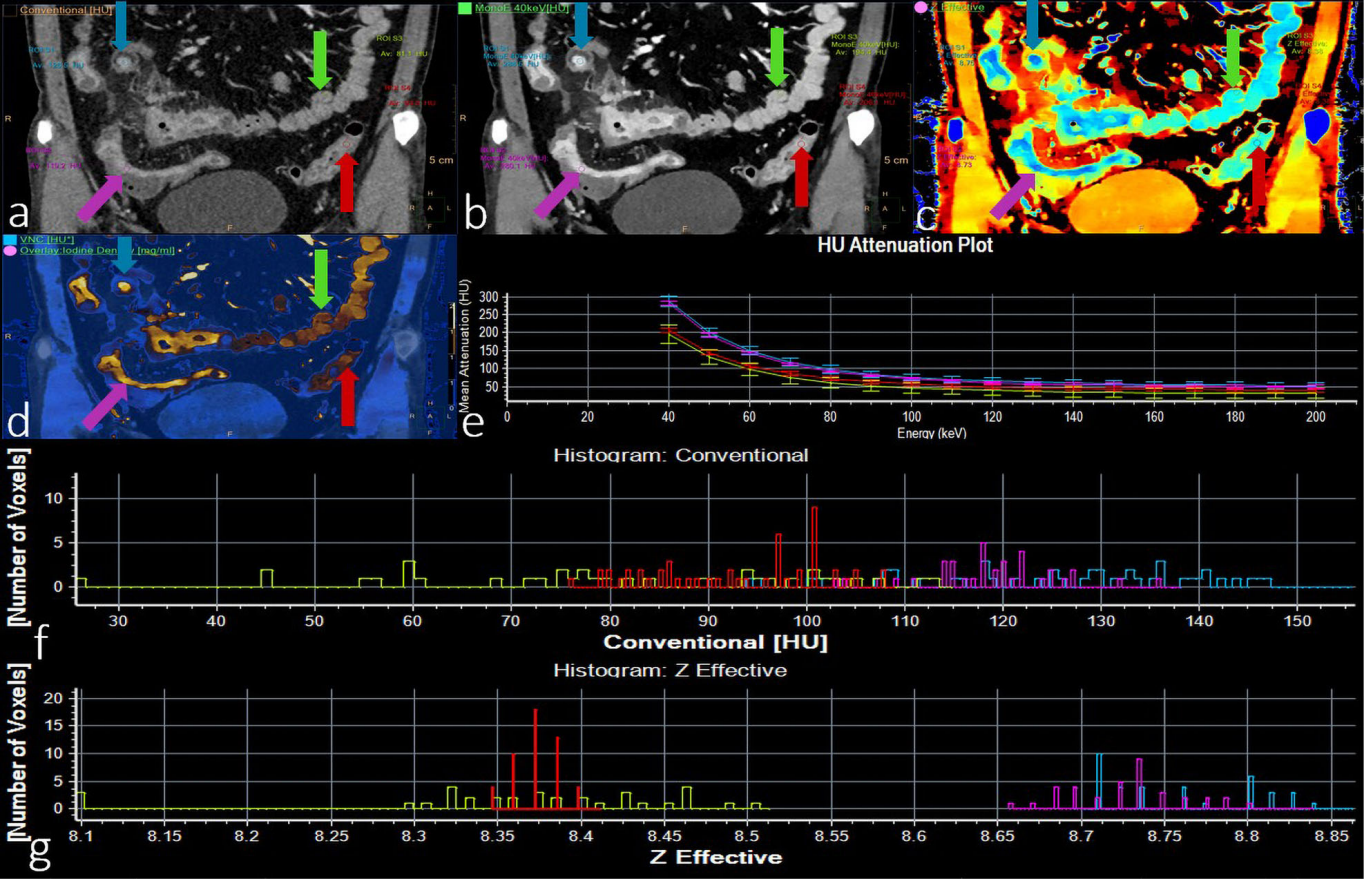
A 43-year-old male patient with CD (Montreal Type A3L3B3, CDAI score 389.55). Arterial phase CT and postprocessing images: a conventional images; b 40 keV images; c
